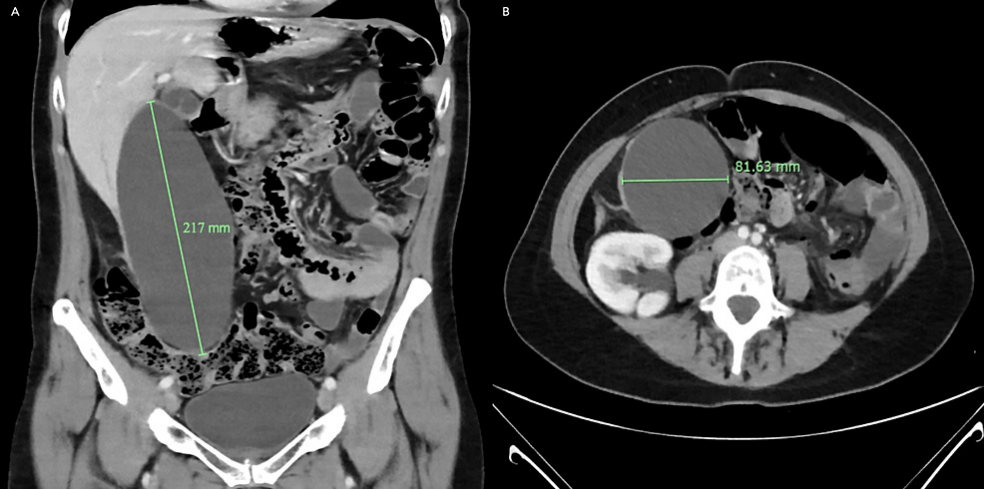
CT images demonstrating a significantly distended thin-walled gallbladder with a (A) maximal cephalocaudal extension of ~217mm and (B) maximal transverse diameter of ~81mm.
